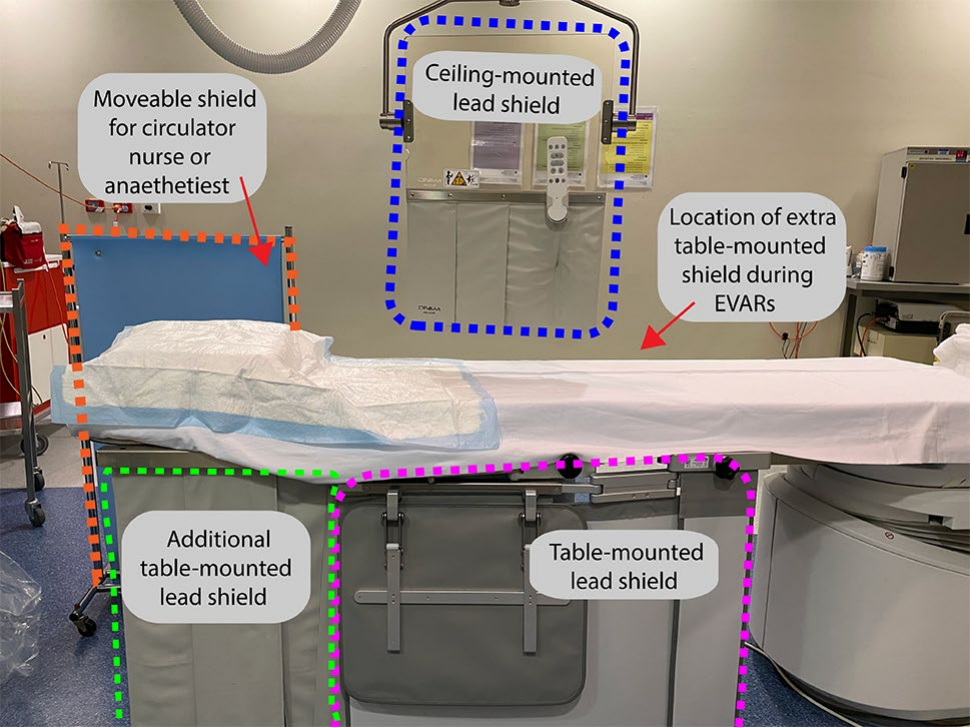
Typical room setup and shielding option including a mobile shield (blue) that can be altered depending on staff position in the room and an adjustable ceiling mounted lead shield to be positioned between the operator and the x-ray detector. *The table mounted shield used on the opposite side of the table for EVAR are the same style as those visualized in the figure
